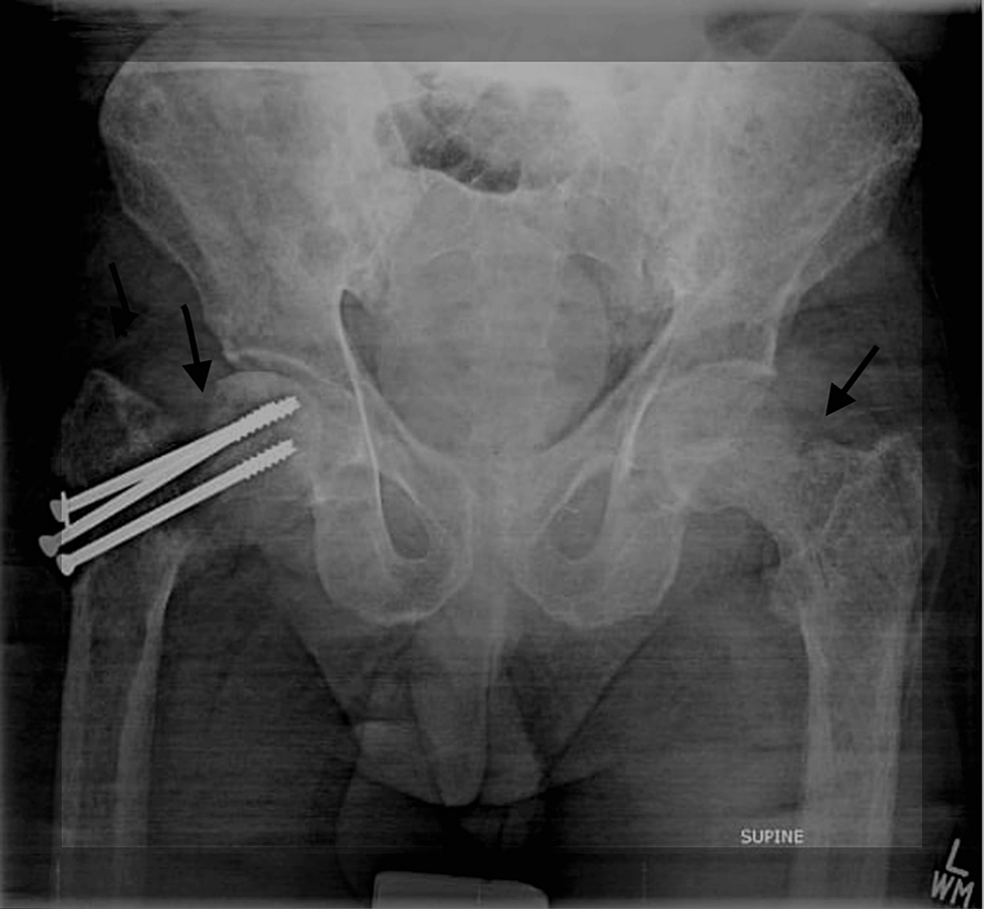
X-ray pelvis anteroposterior view. Visualized bones show a significantly reduced bone density. Suspicion of undisplaced left femoral neck fracture.
